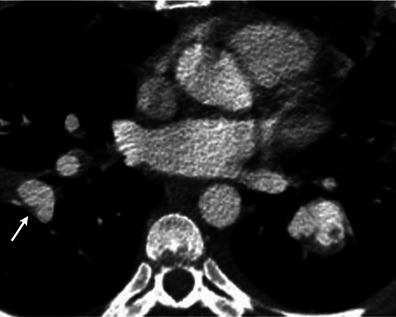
Contrast-enhanced axial CT image demonstrates aneurysmal formation with irregular thick walls in the segmental branches of right and left lower lobe pulmonary arteries (*arrow*) in this patient with a known infected aneurysm. These findings were new compared with prior chest CT
